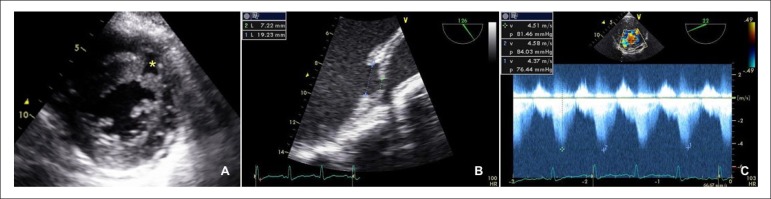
Echocardiographic images of the VSD. Panel A: VSD located in the mid
